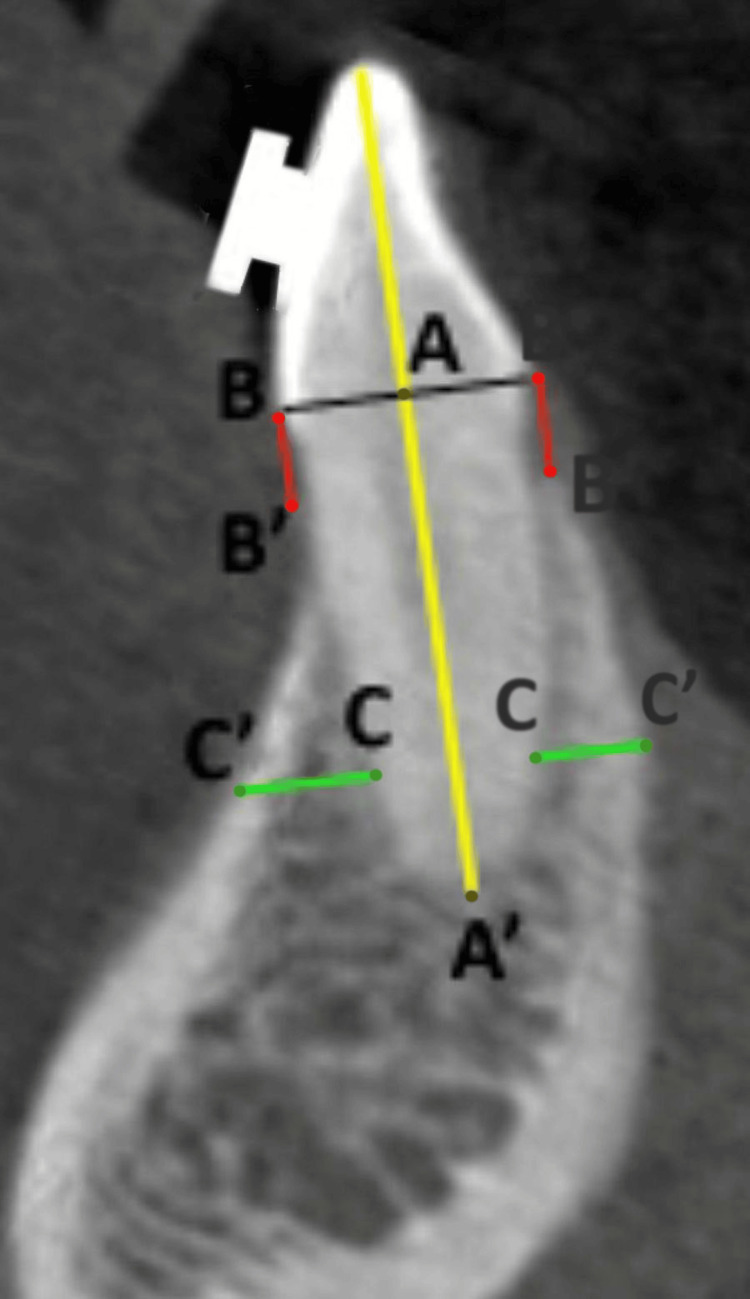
Cone-beam computed tomography image of mandibular central incisor showing measurement of root length (A-A') from the cementoenamel junction to the root apex along the long axis of the tooth, alveolar bone height (B-B') from the cementoenamel junction to the crest of the alveolar bone, and alveolar bone width (C-C') at 3 mm from the apex of the tooth on lingual and labial side. Measurements were done on both labial and lingual aspects. Original image from the case study.
